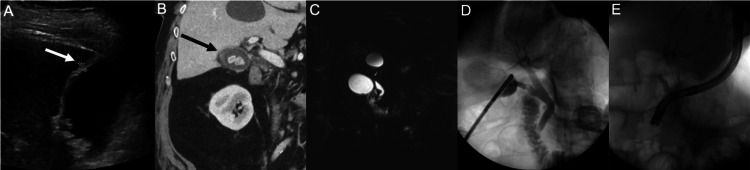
(A) Abdominal ultrasonography showing a slightly thickened gallbladder wall with gallstones (B) Contrast-enhanced abdominal computed tomography scan revealing cholelithiasis with thickening of the gallbladder wall (C) MRCP (D) IOC (E) Fluoroscopy MRCP: magnetic resonance cholangiopancreatography; IOC: intraoperative cholangiogram
